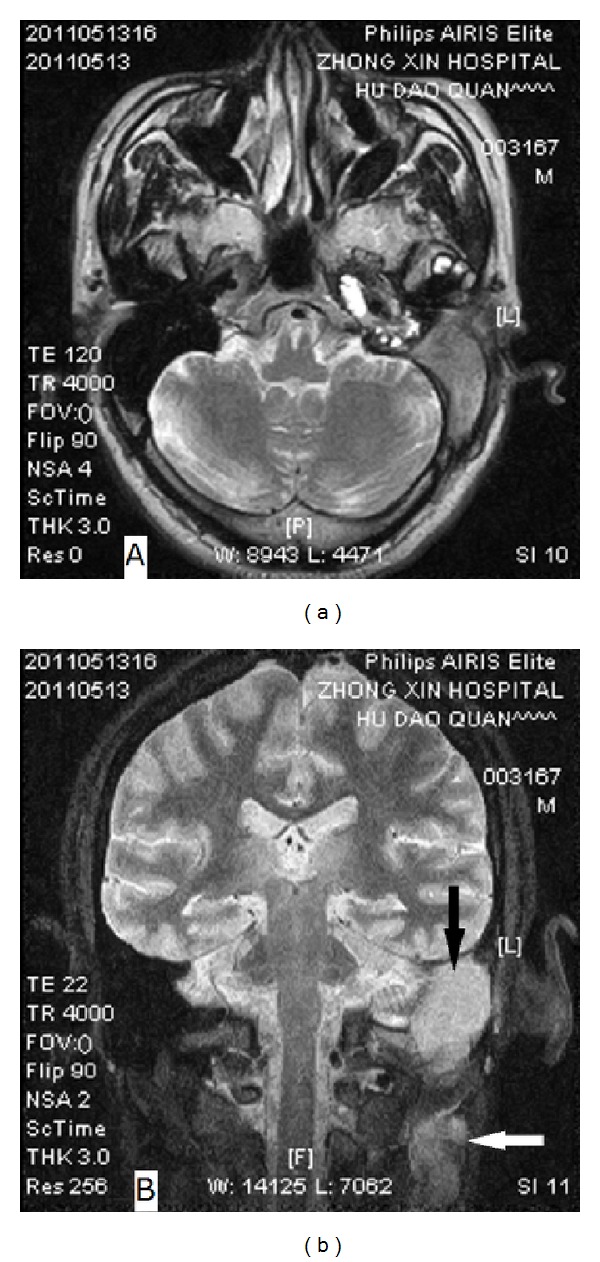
MR images of Case 2. (a) T2-weighted axial image shows a hypointensity lesion in the left mastoid; (b) PDW coronal image demonstrates two nonhomogenous and slightly high-signal-intensity mass in the left mastoid (black arrow) and ipsilateral neck (white arrow), respectively.
